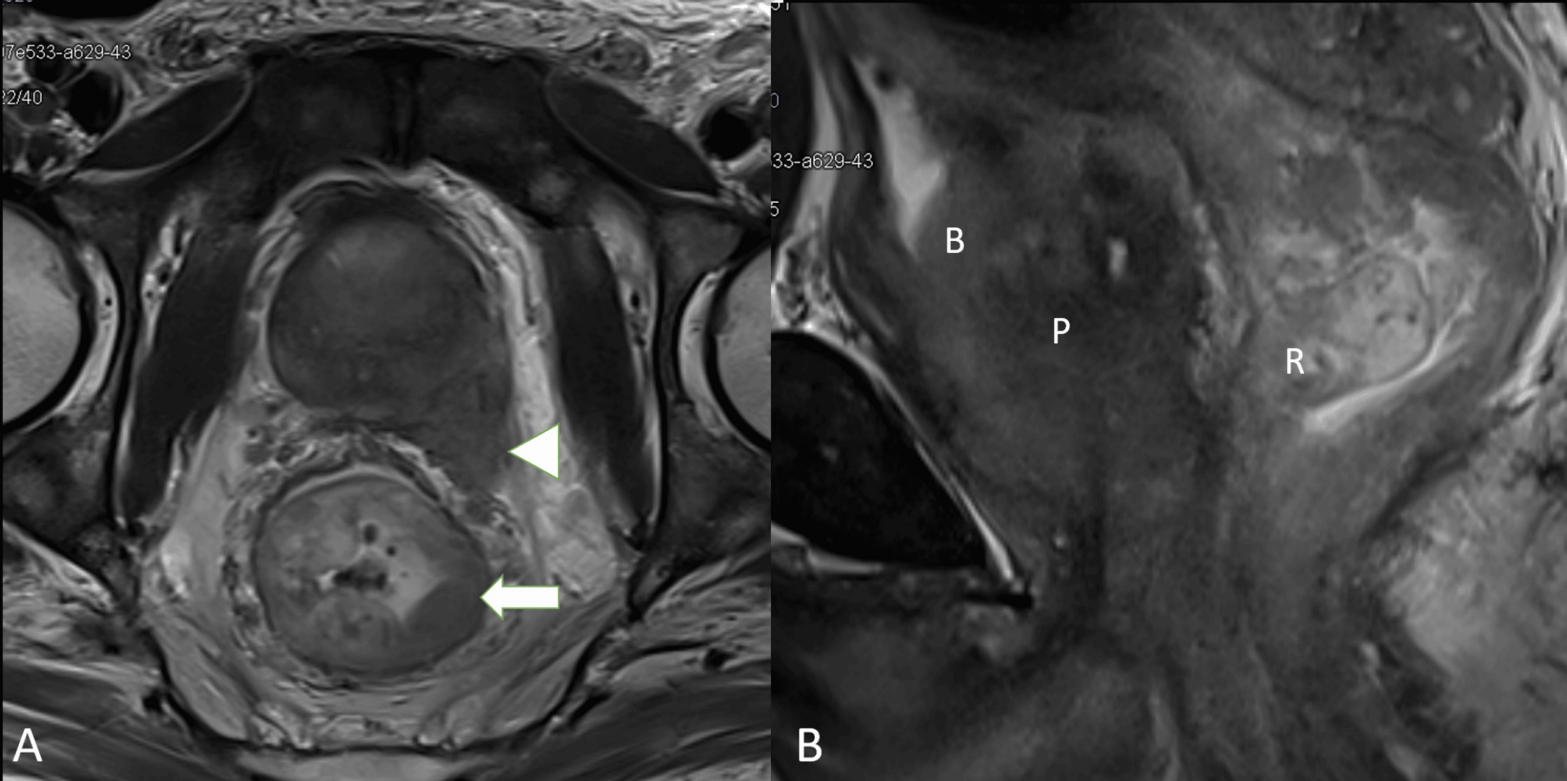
T2W MRI T2W MRI axial and sagittal views (A and B) show irregular circumferential rectal thickening (arrow) with a few suspicious mesorectal lymph nodes. Prostatic mass invades the left seminal vesicle, neurovascular bundle, and urinary bladder (arrowhead), with no direct invasion into the rectum. T2W MRI: T2-weighted magnetic resonance imaging, P: prostate, B: urinary bladder, R: rectum
